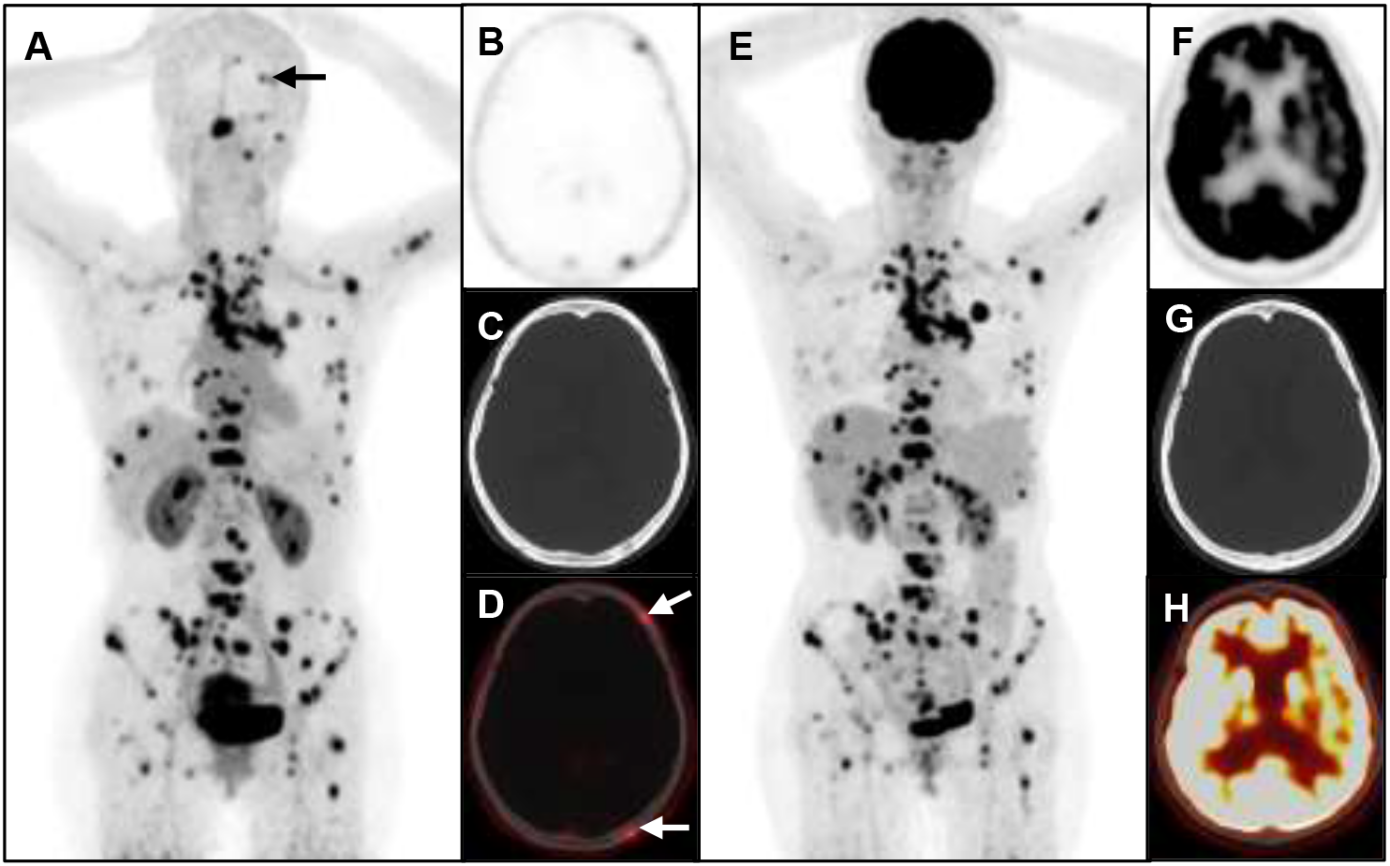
A 53-year-old woman with metastatic lung cancer underwent cancer restaging imaging. ^68^Ga-FAP-2286 shows higher uptake in skull lesions (A-D), straight arrow, SUVmax 3.93).^18^F-FDG shows no uptake in skull lesions (E-H).
